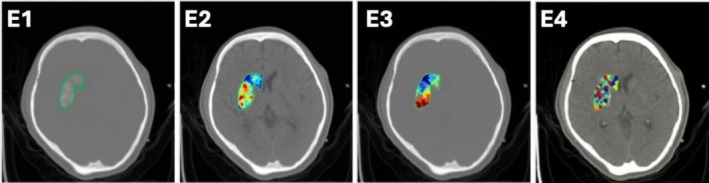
(E) A 50‐year‐old male patient with cerebral hemorrhage. (E1) Arterial‐phase CT with lesion delineation. (E2) Nested habitat analysis results. (E3) Intensity‐based k‐means clustering results. (E4) Super pixel clustering results.
